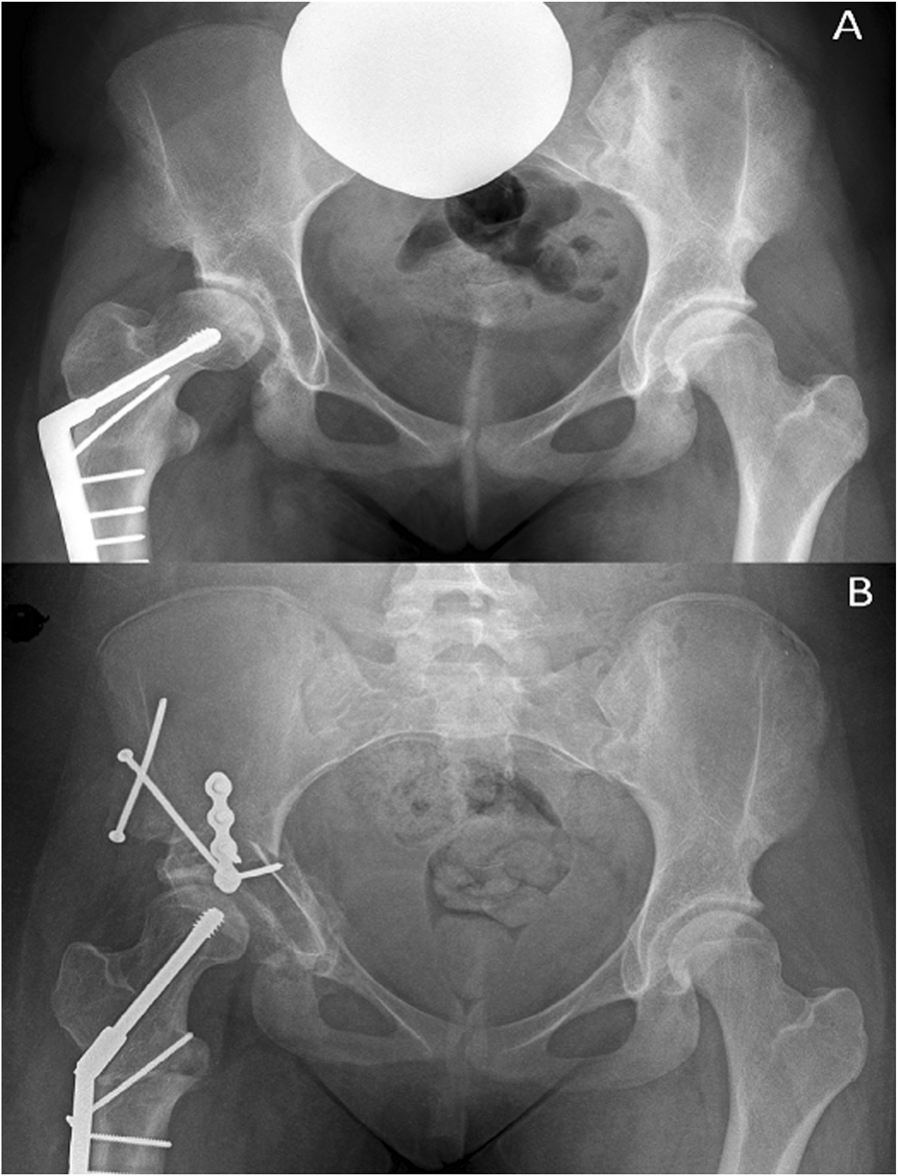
Example of preoperative (A) and postoperative (B) radiographs following Interlocking Pelvic Triple Osteotomy. Note in the preoperative film evidence of a previous proximal femoral varus osteotomy. In the postoperative film, the acetabular orientation has been corrected, and the proximal femur has been repositioned to a physiological orientation.
